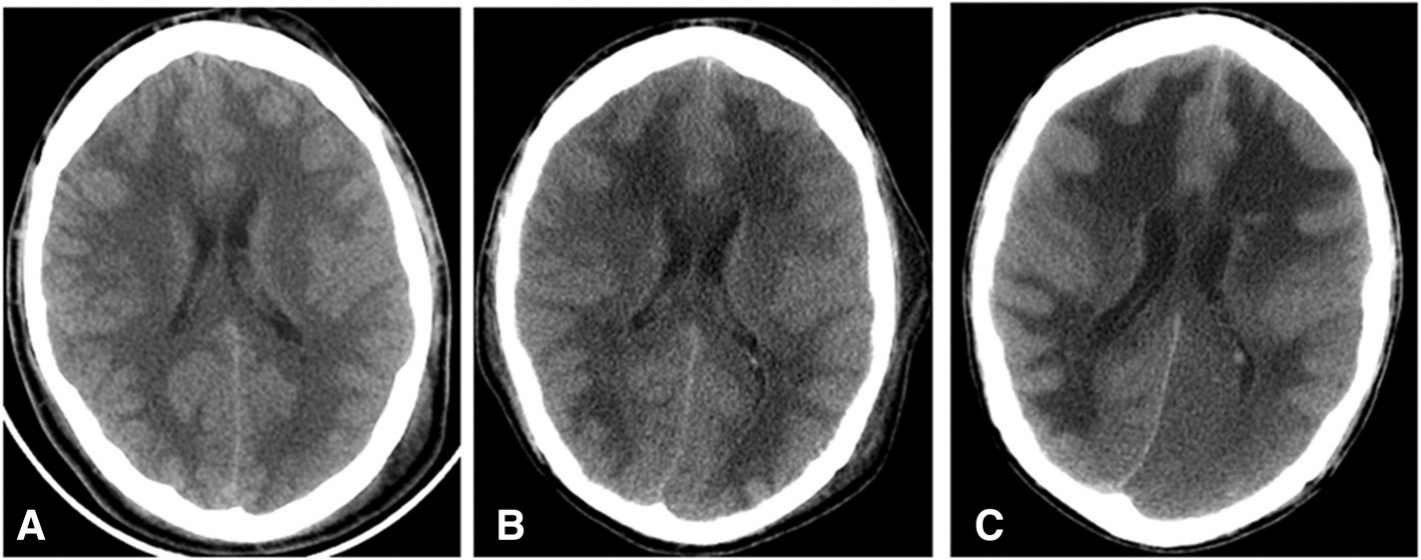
Brain CT scans throughout the hospital stay. A) admission CT scan showing an unremarkable brain parenchyma with preserved grey-white matter demarcation. There was also small left frontal scalp hematoma (not shown). B) A follow-up CT showing decreasing attenuation of the white matter diffusely. C) CT done 3 weeks of admission following patient clinical deterioration revealing evidence of progressive diffuse hypoattenuation the cerebral white matter, basal ganglia and thalami bilaterally. There is left occipital gray-white matter hypodensity consistent with infarct, explained by trantentorial herniation trapping the left posterior cerebral artery against tentorium cerebelli.
